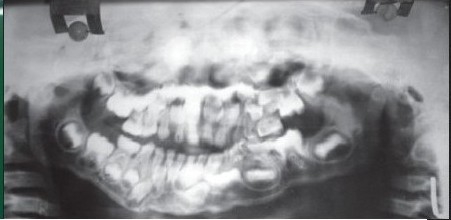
Preoperative orthopantomogram showing right sided temporomandibular joint ankylosis
